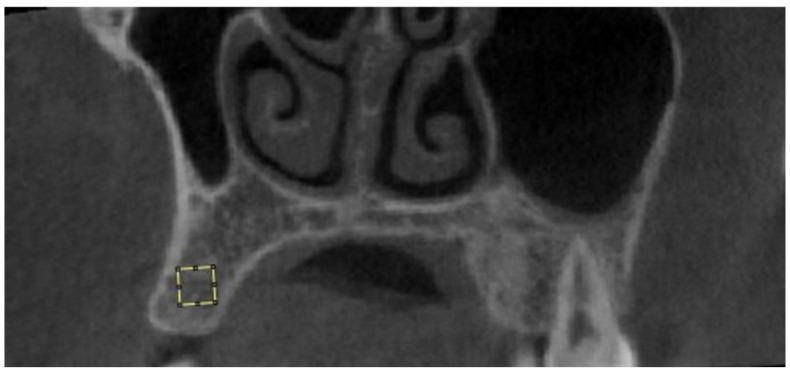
Selection of 20 × 20 pixel ROI on a CBCT image.
